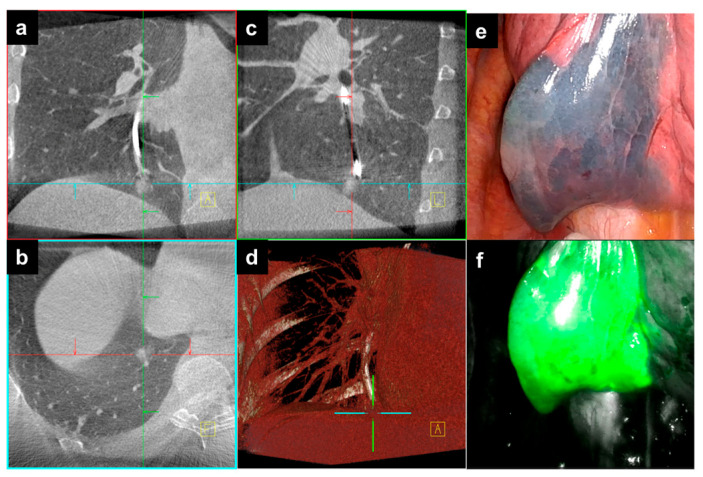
Intraprocedural cone-beam computed tomography (CBcT) utilized in concert with robotic bronchoscopy to localize a 15 mm right lower lobe ground-glass nodule and instill local methylene blue and indocyanine green. (a): coronal, (b) axial, (c) sagittal, and (d) real-time 3D reconstruction. Intraoperative appearance of (e) methylene blue and (f) indocyanine green.
